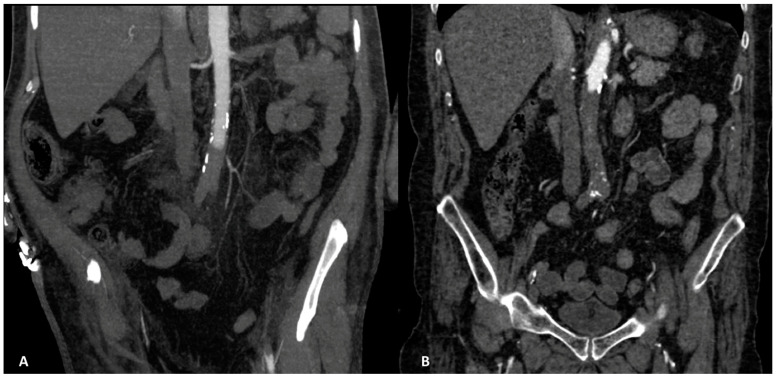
(A). CT angiography, coronal planes, arterial phase, MIP reconstruction. Aortic complete occlusion in the subrenal tract. Thrombosis located also in the left renal artery and extended until left common femoral artery can be noted (in our study found in 3/11 patients). (B). CT angiography, coronal planes, arterial phase. Aortic complete occlusion in the infrarenal tract, located at the origin of the right renal artery.
